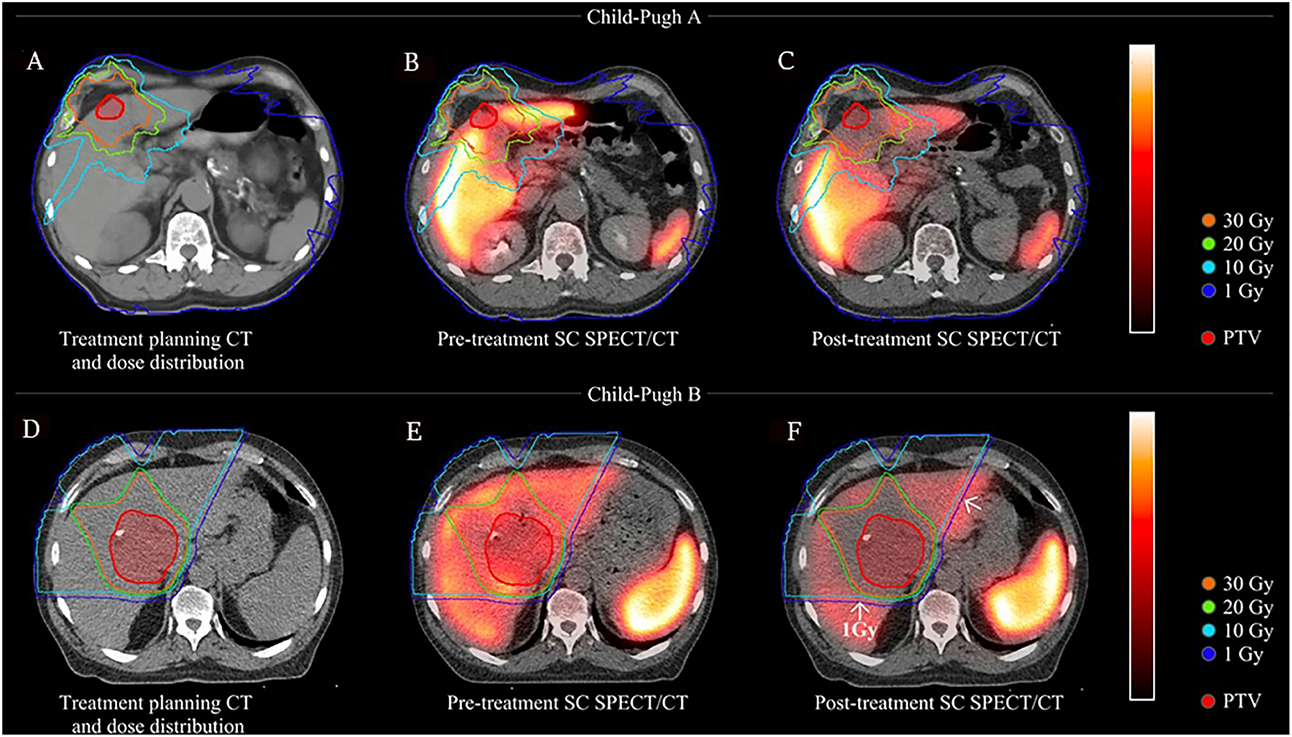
Visualization of spatial functional liver loss with SC SPECT/CT. 1st row (A-C): CP-A patient; 2nd row (D-F): CP-B patient; 1st column (A and D): radiation treatment plans with isodose lines; 2nd column (B and E): radiation treatment plans overlaid with pre-treatment SC SPECT/CT scans; 3rd column (C and F): radiation treatment plans overlaid with post-RT SC SPECT/CT scans demonstrating a higher threshold for function liver loss for the CP-A patient at 30 Gy and above (C) compared to the CP-B patient at 10 Gy and above (F). SC: Sulfur colloid; CP: child-pugh; RT: radiotherapy.
